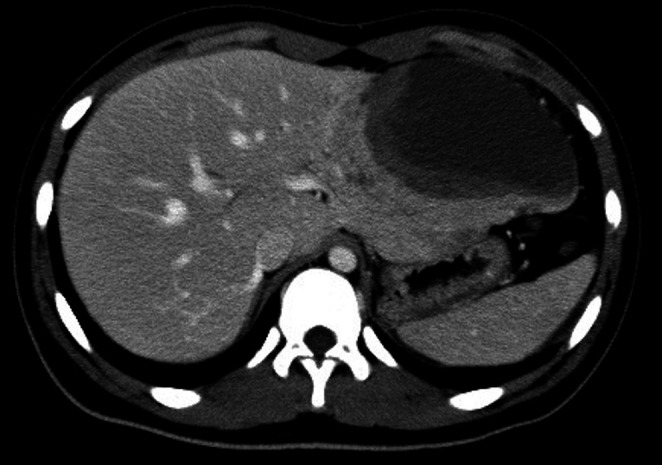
A contrast‐enhanced CT of the abdomen revealed a low‐attenuation mass (12 × 8 × 6 cm) in the left lateral lobe of the liver with poor enhancement that was observed in the lateral segment of the left hepatic lobe.
